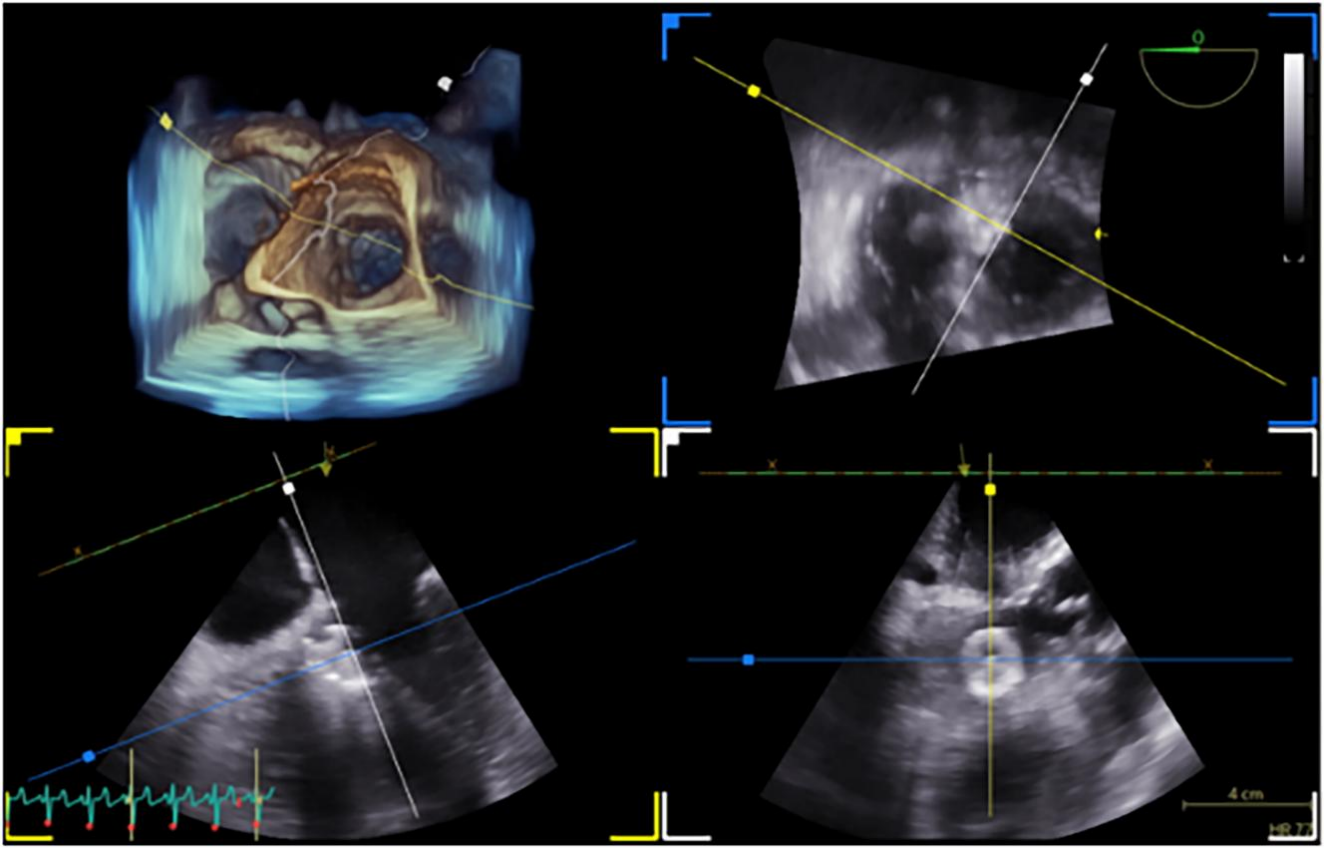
Intraprocedural transoesophageal echocardiogram was after the closure of the ventricular septal rupture.
